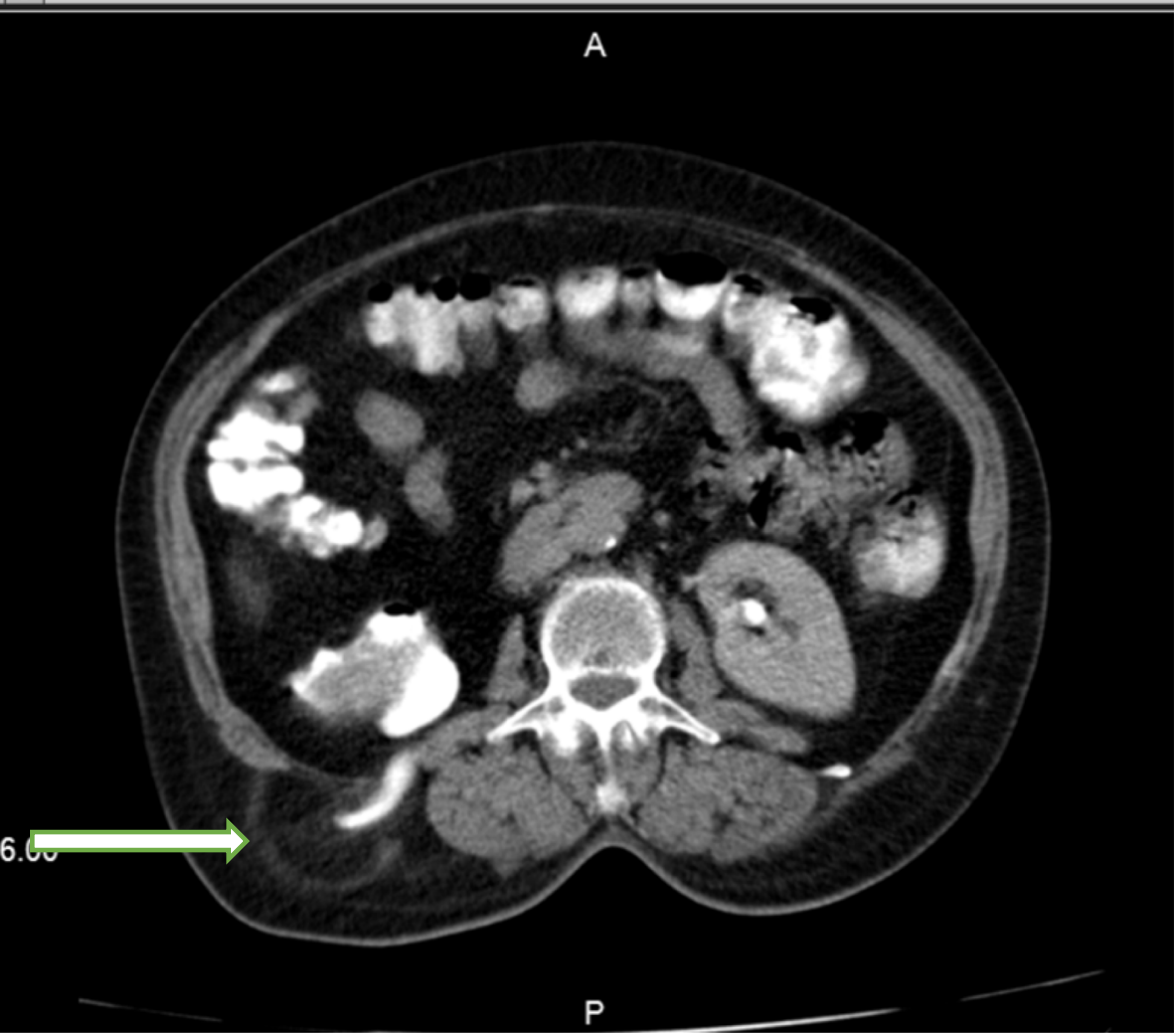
Spiral CT scan with intravenous (IV) contrast: herniated sac containing right ureter (white arrow)
